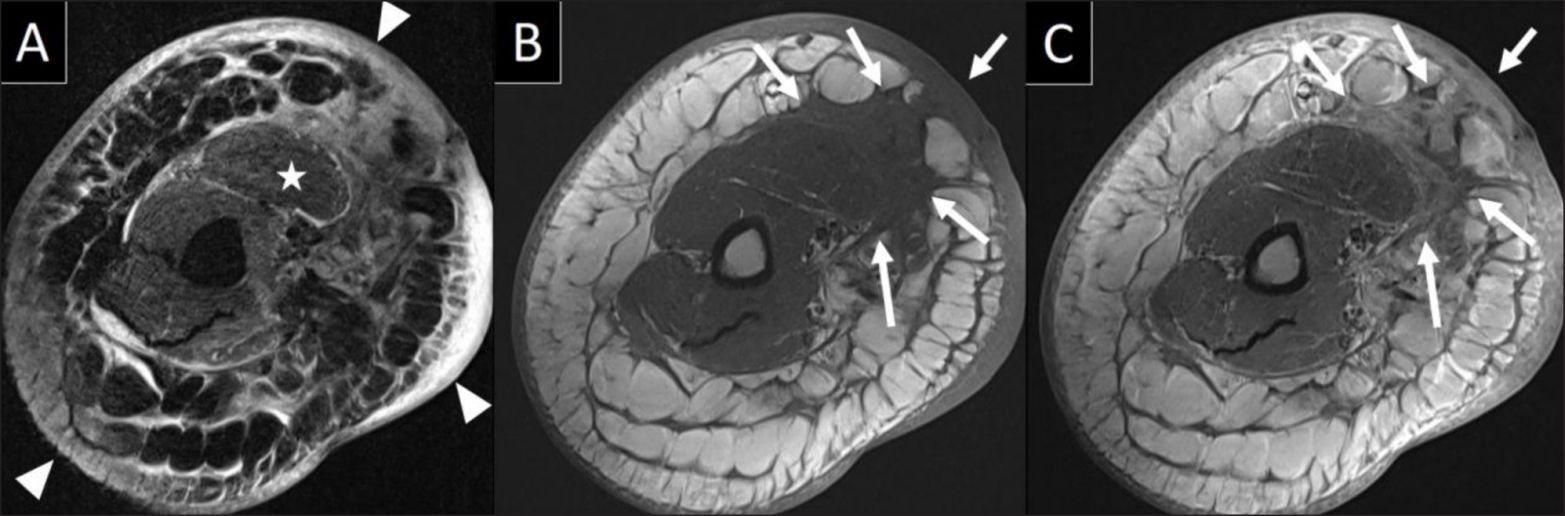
MRI of the right upper arm: axial TIR (a), T1 before (b) and T1 after (c) intravenous contrast. Diffuse thickening of the cutis and subcutis (arrowheads in 1a) as well as muscle edema (asterisk in 1a) can be seen. There is an amorphous heterogeneous enhancing mass (arrows in 1b and 1c) extending from the superficial cutis to the biceps muscle, with spicular infiltration reaching the neurovascular bundle.
